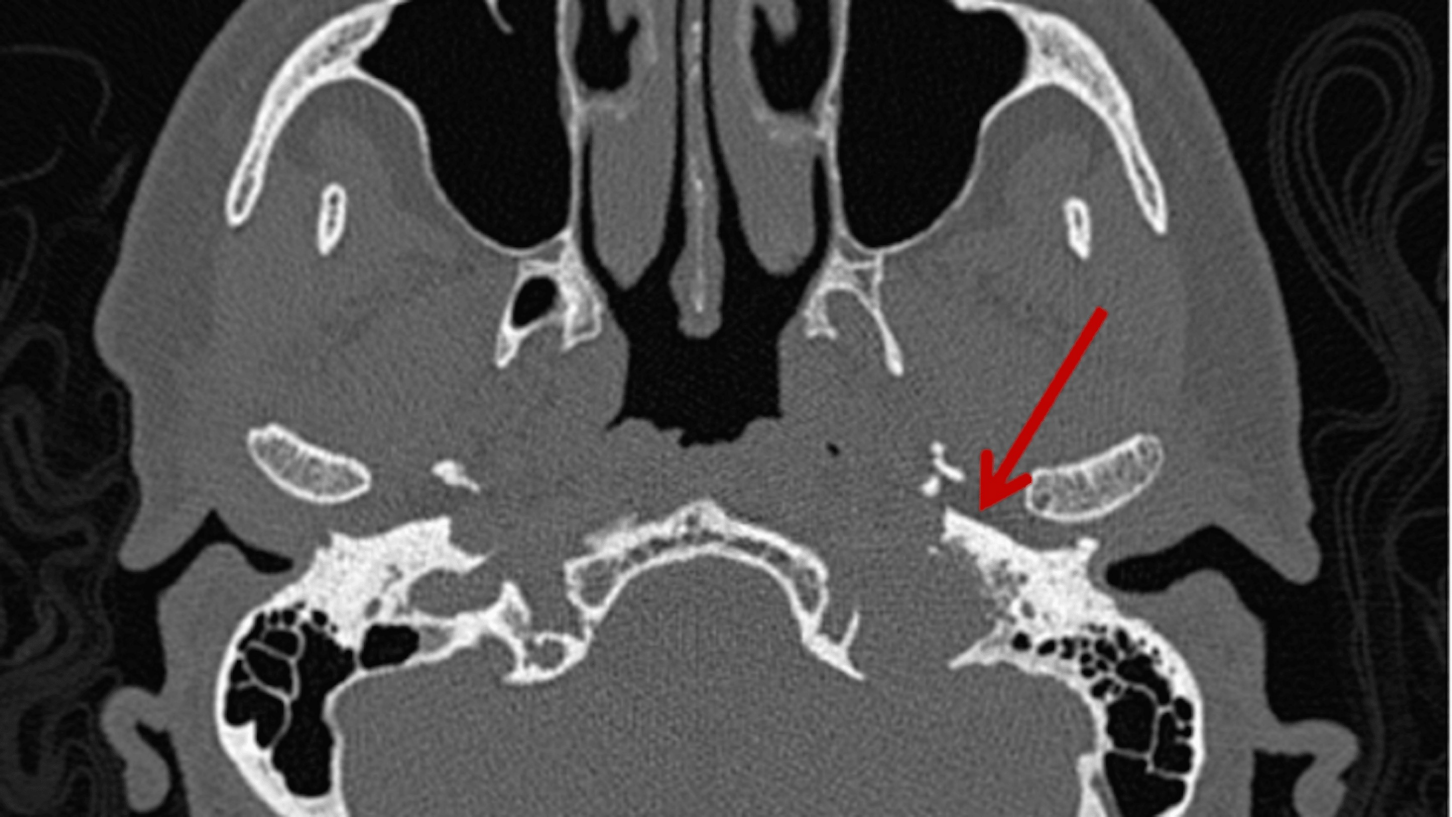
Axial non-contrast-enhanced computed tomography scan of the skull base at the level of the jugular foramen. Erosion of the left jugular foramen is evident (red arrow), with mild asymmetry of the jugular bulbs, suggesting an expansive lesion in this region consistent with a jugular paraganglioma.
